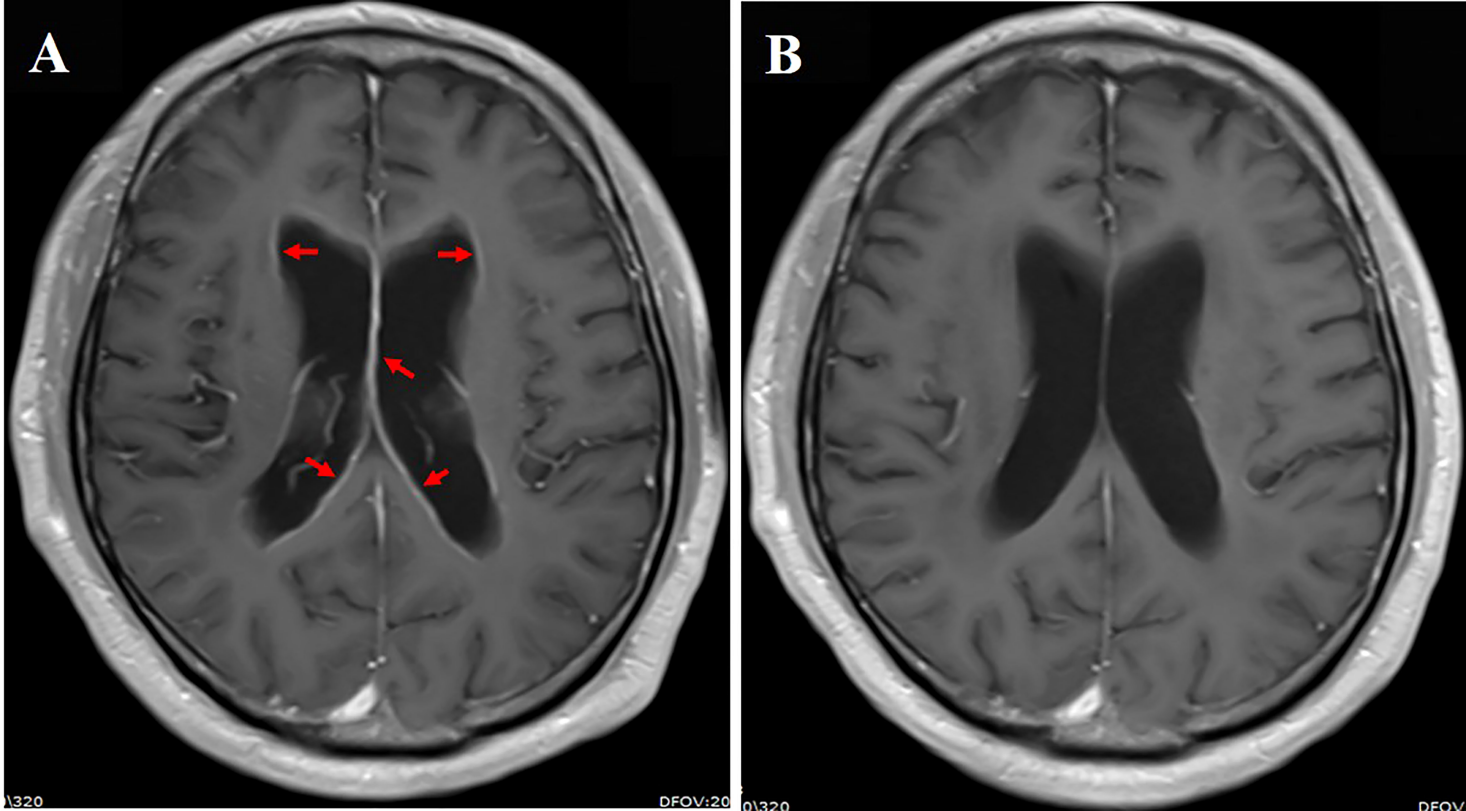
(A) Cranial enhanced MRI showing linear enhancement of ventricular ependyma (red arrow); (B) linear enhancement of leptomeninges regressed 2 months after aumolertinib plus bevacizumab treatment.
